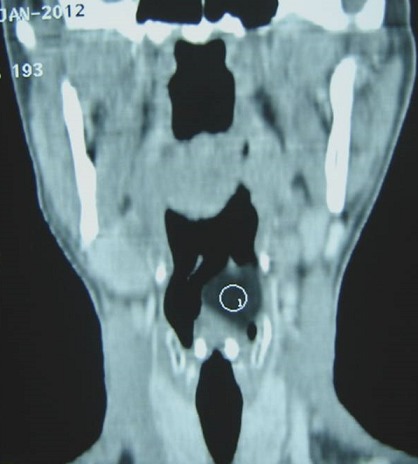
Coronal reformation CT image: showed that this lesion (circle) is exerting a compression on the pyriform sinus and the larynx, with respect to the neck vessels
